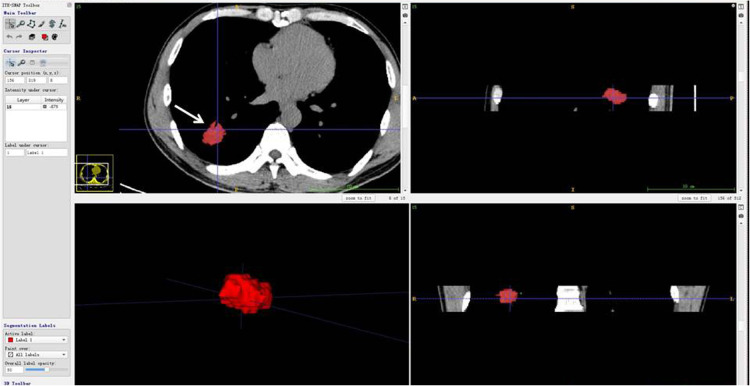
CT scan of the patient (woman, 77 years old) showing a solid nodule in the post-basal segment of the right lower lobe, which proved to be lung infiltrating adenocarcinoma. The 3-dimensional imaging (all slices) of segmentation was imported into AK software (Artificial Intelligence Kit, Version 3.3.0, GE Healthcare) (13) for feature extraction. The imaging presented unenhanced imaging: the left upper figure was cut into images (arrow), the left lower figure was cut into whole nodules, and the upper and lower figures on the right are vertical plane and coronal plane images of the cut nodules.
